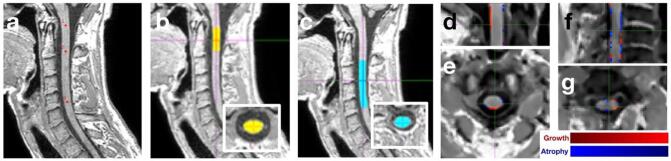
Spinal cord MRI processing using SCT and GBSI. Reference points were manually set at C1, C2 and C5 on sagittal spinal cord scans (a). Then, masks of C1-2 (b) and C2-5 (c) spinal cord levels were obtained from spinal cord images acquired at each time point using the *DeepSeg* tool within the SCT (version 4.0) (sagittal planes are presented, with axial planes in the inset). The probabilistic boundary-shift region-of-interest was then adaptively estimated from baseline and follow-up spinal cord segmentations and the GBSI integral was ultimately computed for C1-2 (d for sagittal plane, e for axial plane) and C2-5 (f for sagittal plane, g for axial plane). CSA: cross-sectional spinal cord area; GBSI: generalised boundary-shift integral; MRI: magnetic resonance imaging; SCT: spinal cord toolbox.
